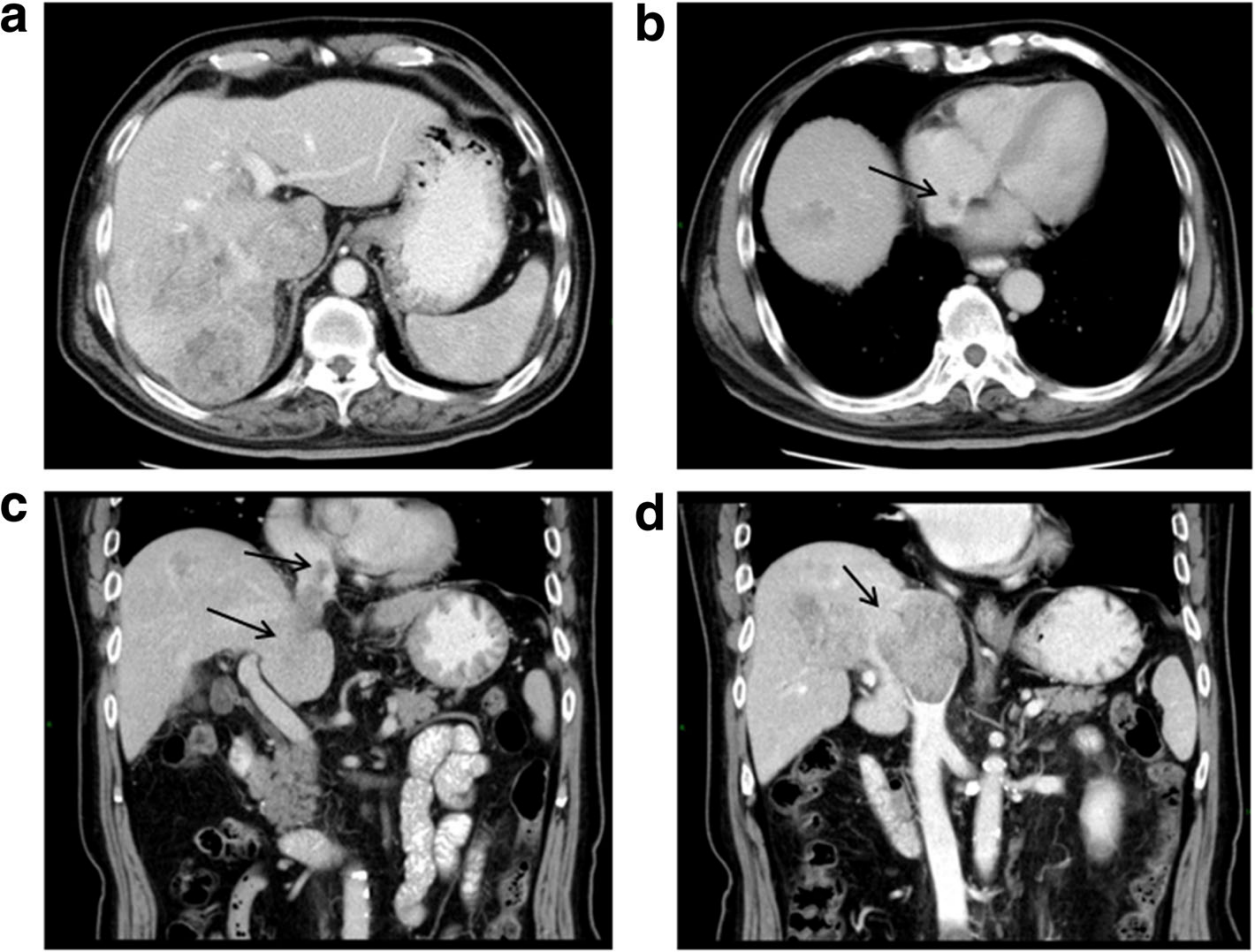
Pre-operative CT scanning. a Enhanced CT of the abdominal region showed multiple tumor nodules at the right lobe of the liver and tumor thrombus in IVC. b Enhanced CT showed an accompanying tumor thrombus in RA (*black arrow*). c, d Enhanced CT showed tumor thrombus in IVC and RA (*black arrow*). CT: computed tomography, RA: right atrium, IVC: inferior vena cava
